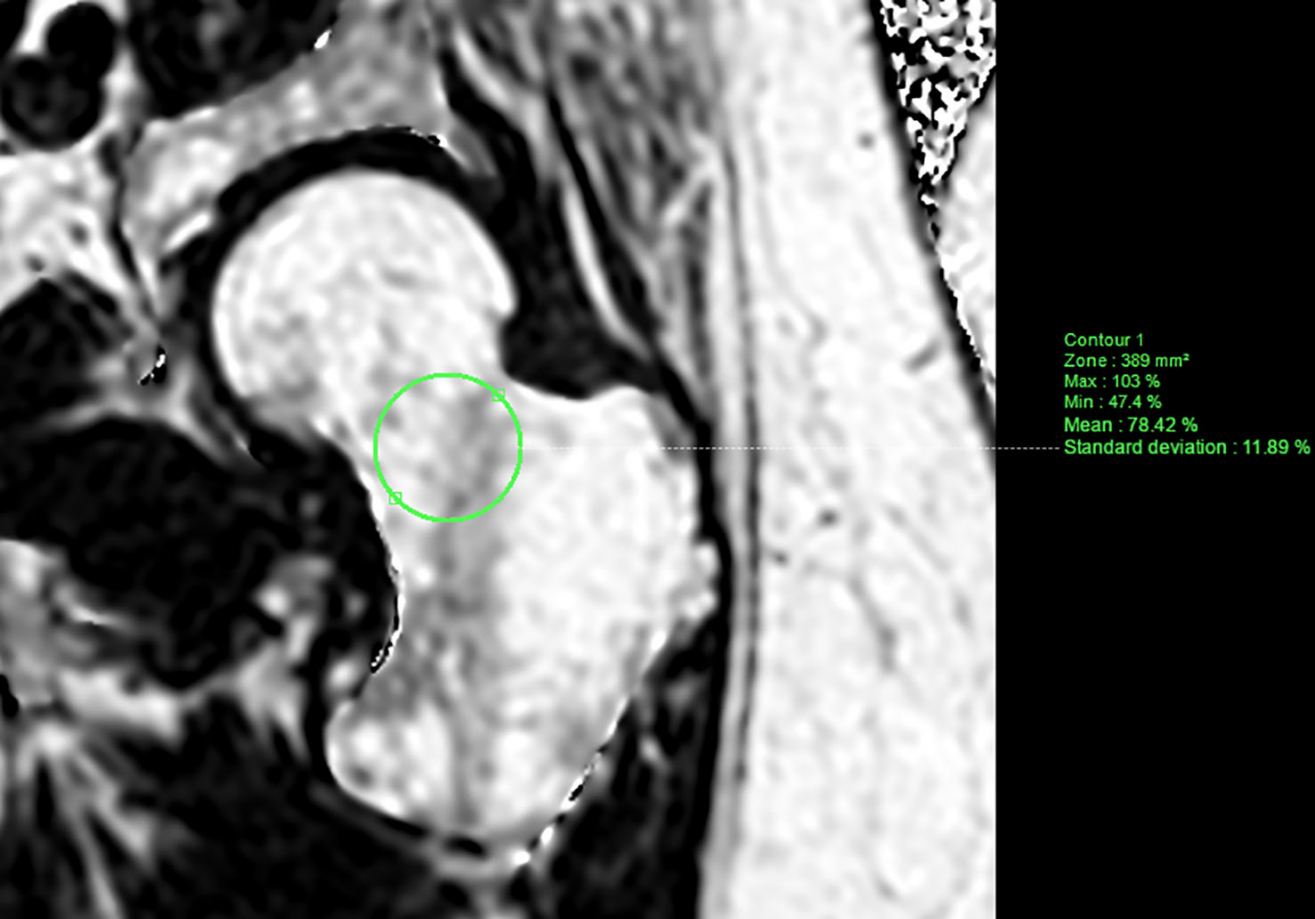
PDFF map of the left (non-dominant) hip computed from a T1-weighted multi-echo gradient echo sequence (mDixon-Quant) acquired in a coronal oblique plane (along the femoral neck axis), from a 51-year old case. Manually segmented ROI (*green*) was placed in the femoral neck avoiding the cortical bone.
